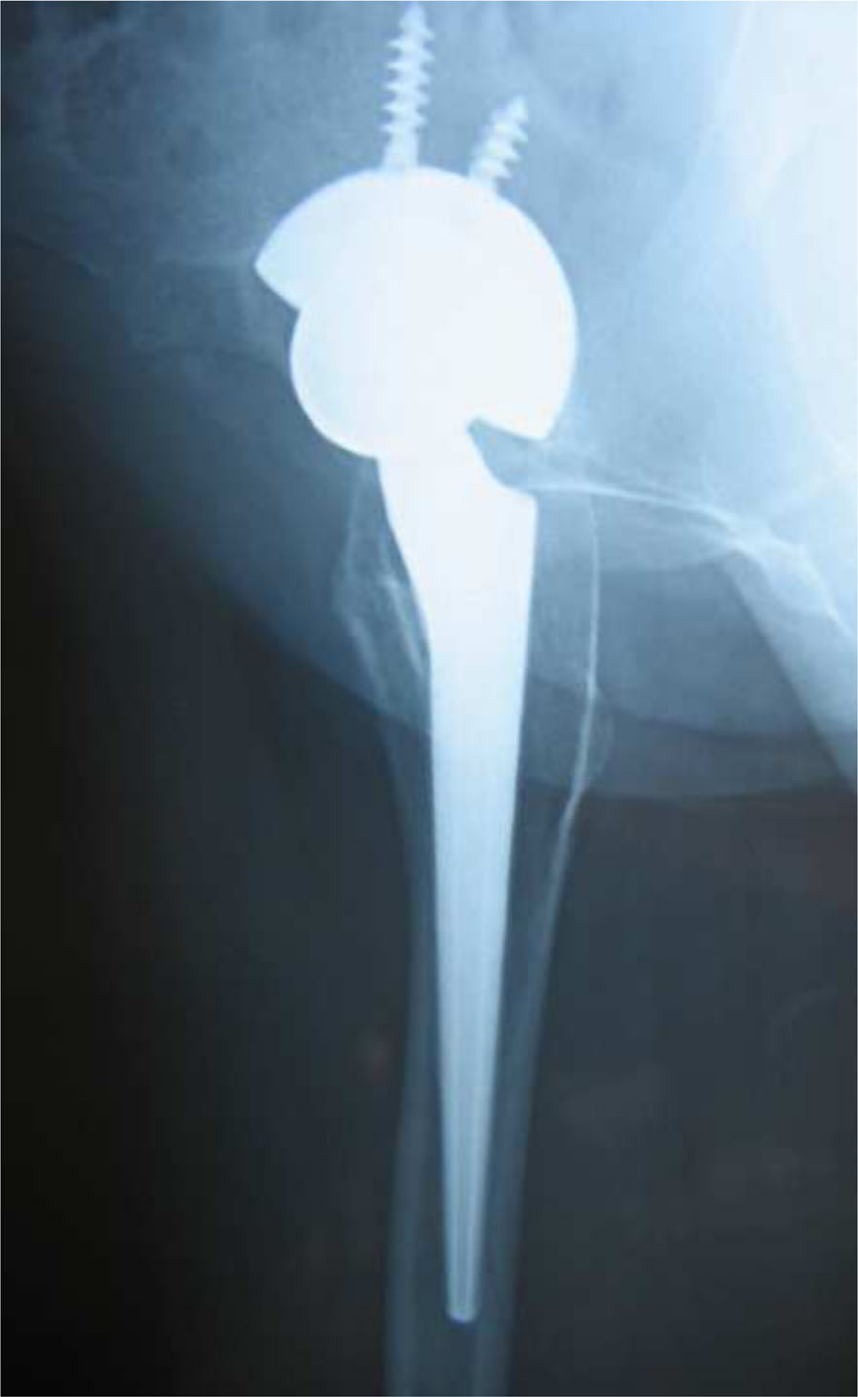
Frog-leg lateral radiograph on the right side at 8 years of follow-up
